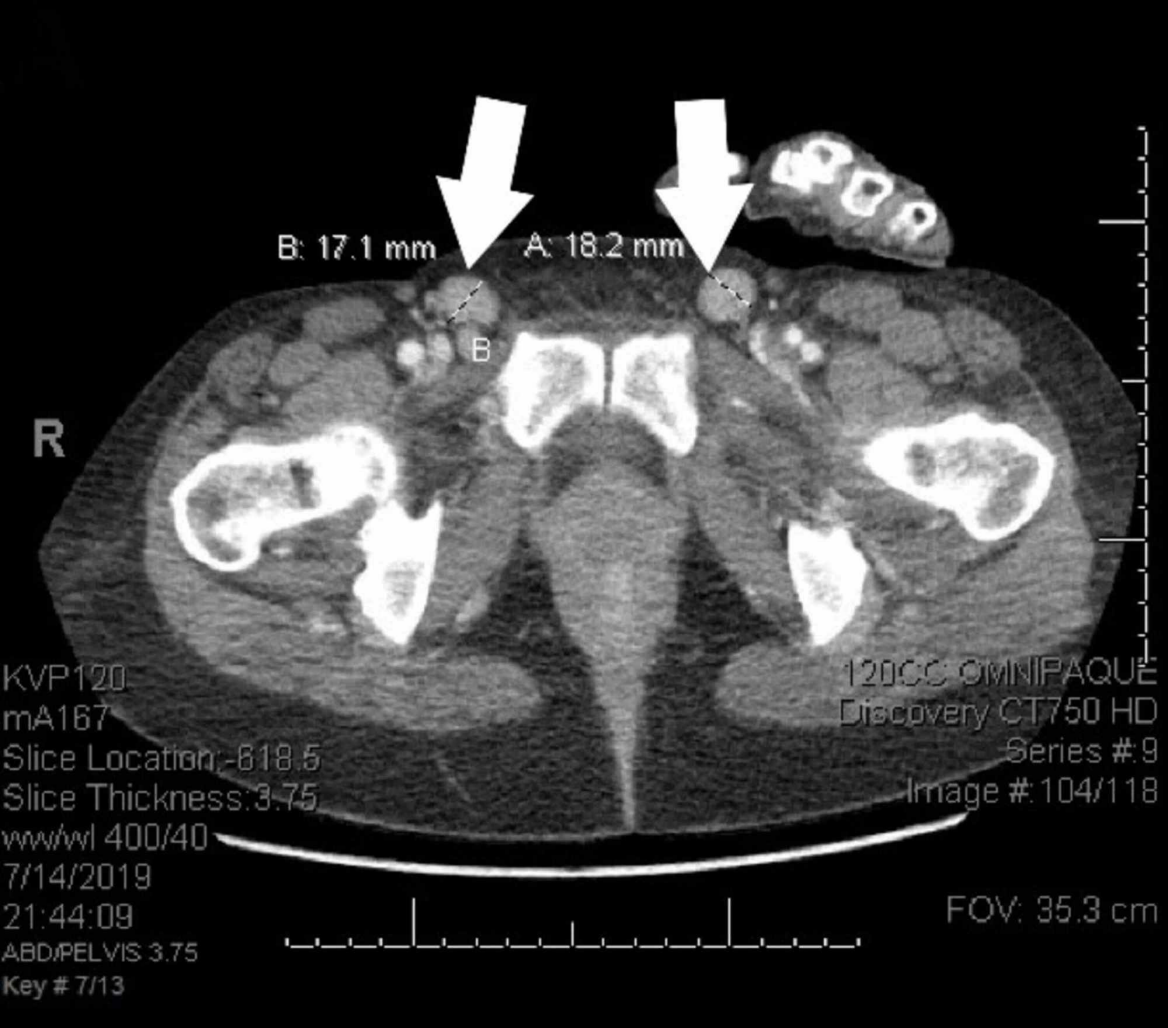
CT scans before treatment The patient’s initial CT scan showed bilateral inguinal lymphadenopathy with 18.2 mm (A) and 17.1 mm diameters (B) CT: computed tomography
